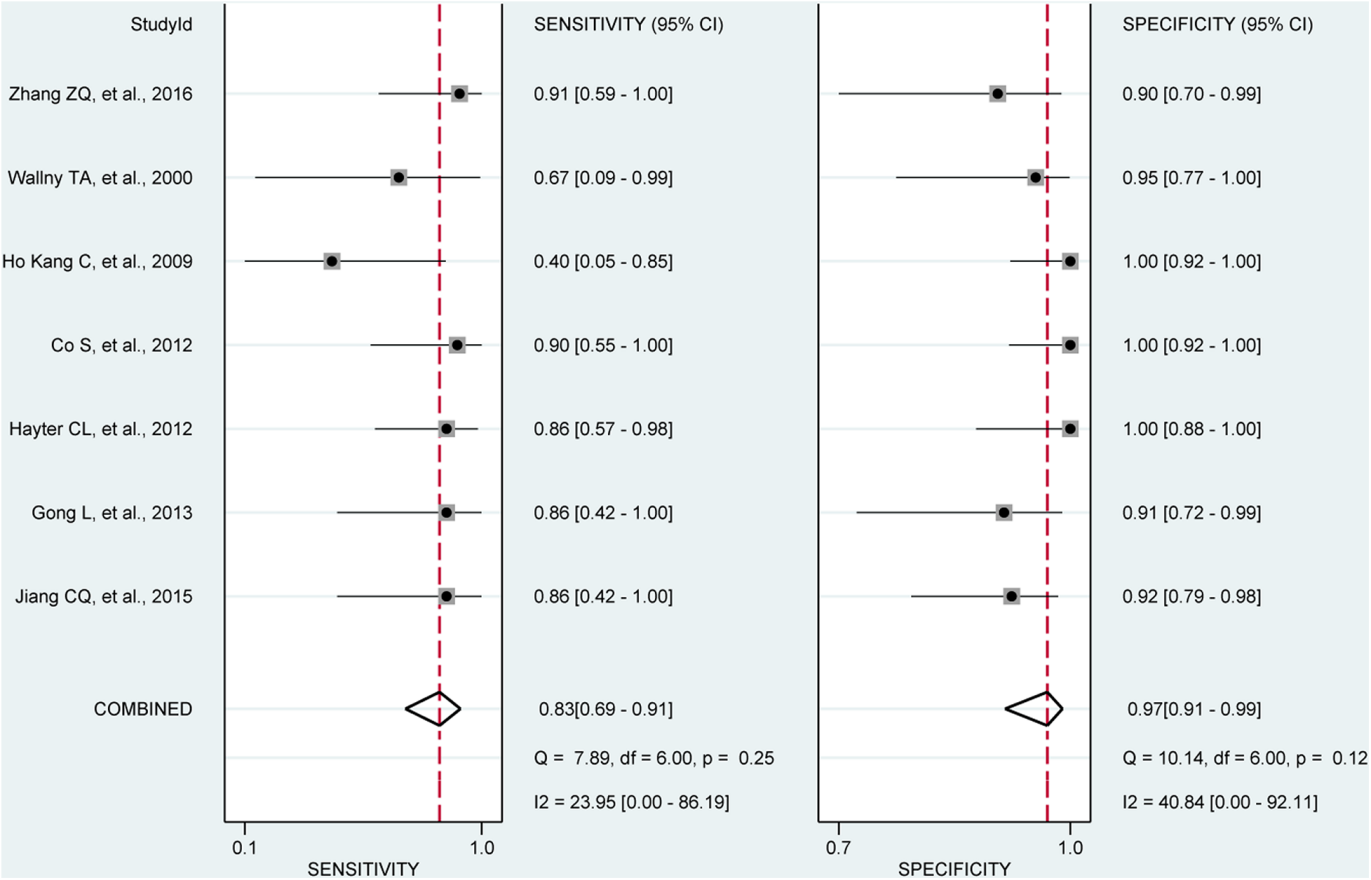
Forest plots of the pooled sensitivity and specificity to diagnose partial-thickness rotator cuff tears with the corresponding 95% confidence region. Three-dimensional sonography for the diagnosis of patients with partial-thickness rotator cuff tear (seven studies, the pooled sensitivity = 0.83, *I*^2 ^= 23.95%; the pooled specificity = 0.97, *I*^2 ^= 40.84%). CI, confidence interval.
